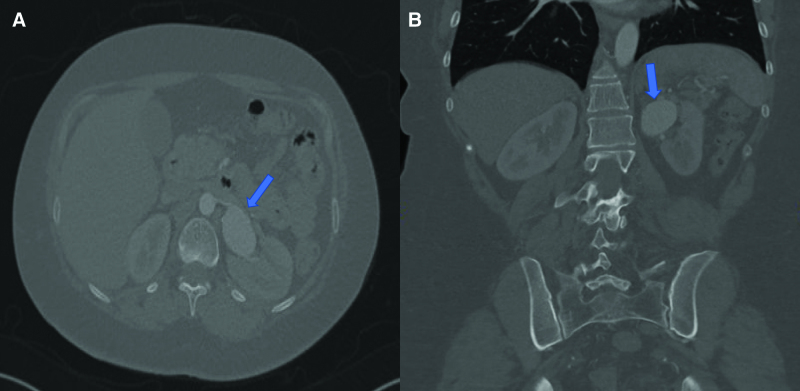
(A) Computed tomography angiography of the renal arteries axial view showed a fusiform aneurysm of the distal left renal artery measuring 4.1 × 2.7 × 4 cm with peripheral calcifications (arrowhead). (B) Coronal view of the fusiform aneurysm of the distal left renal artery.
